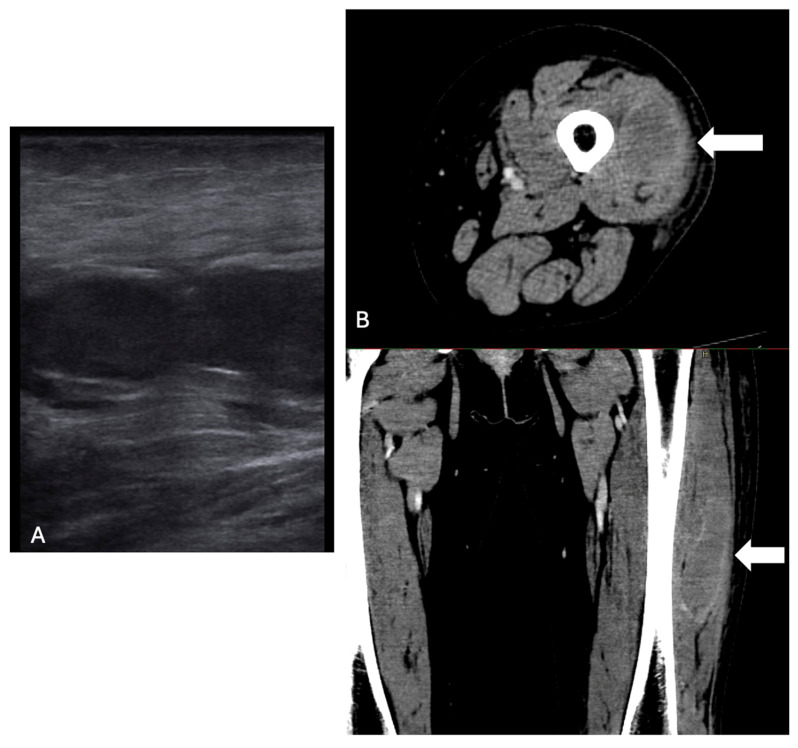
Left lateral rectus muscle hematoma at ultrasound (A) and a computerized tomography (B) scan (indicated with white arrows).
